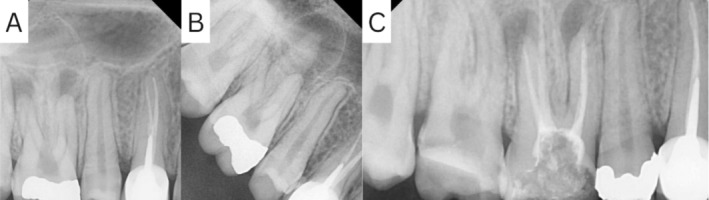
Initial and final radiographs taken by the referring dentist: (A) pre‐operative periapical radiograph; (B) pre‐operative mesial‐angulated radiograph; (C) post‐treatment radiograph after the initial RCT with temporary restoration.
